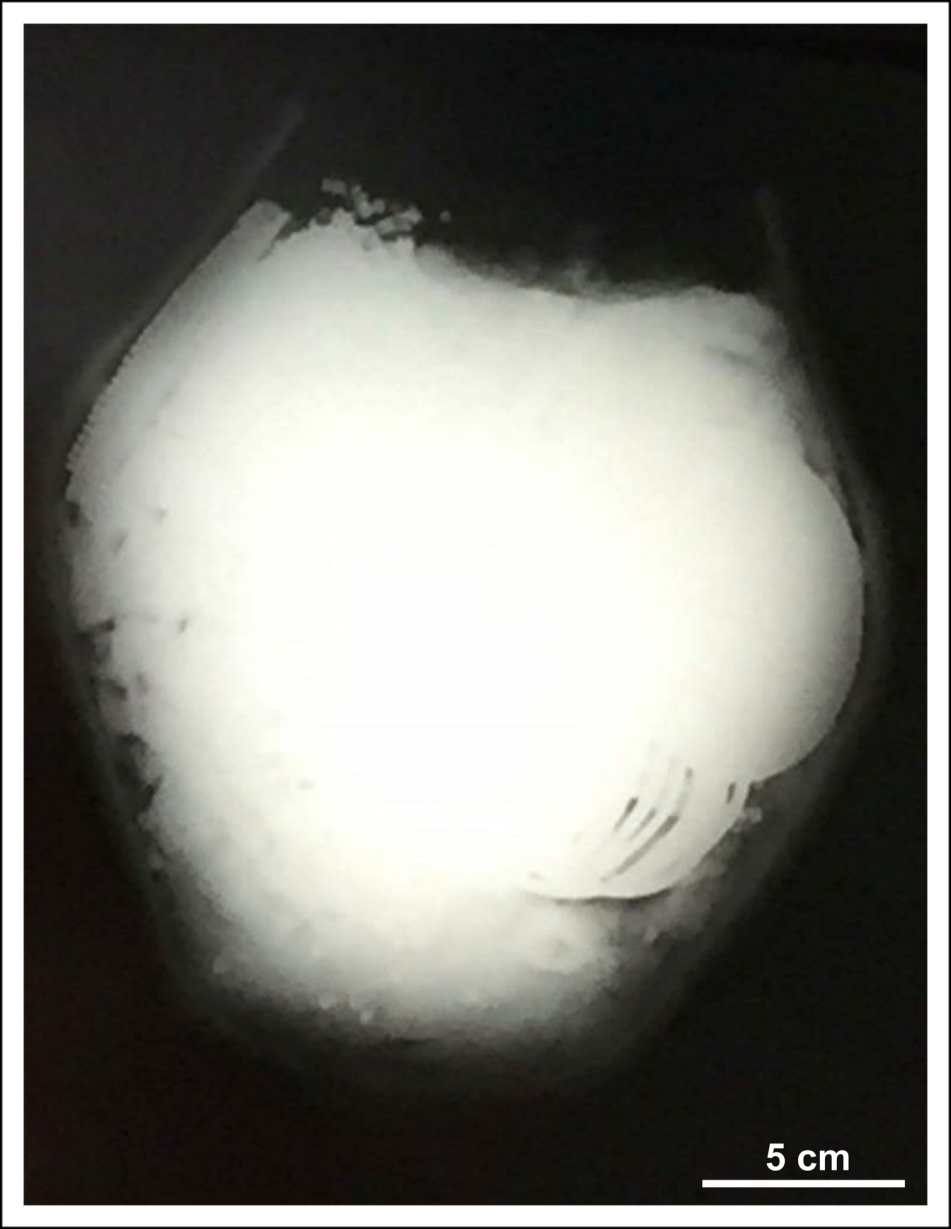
X-Ray image of the hoard.
